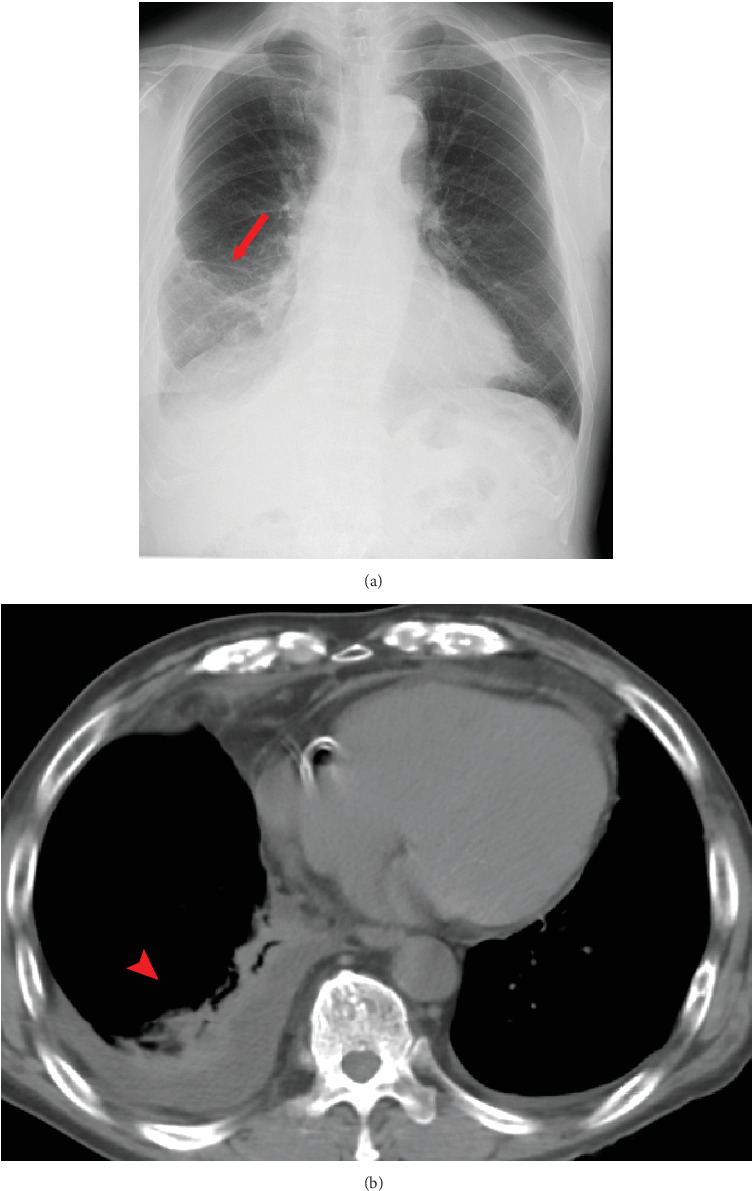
(a) Chest x-ray film and (b) CT image, revealing right pleural thickening, lung volume loss, and pleural effusion in Case 1. CT, computed tomography.
